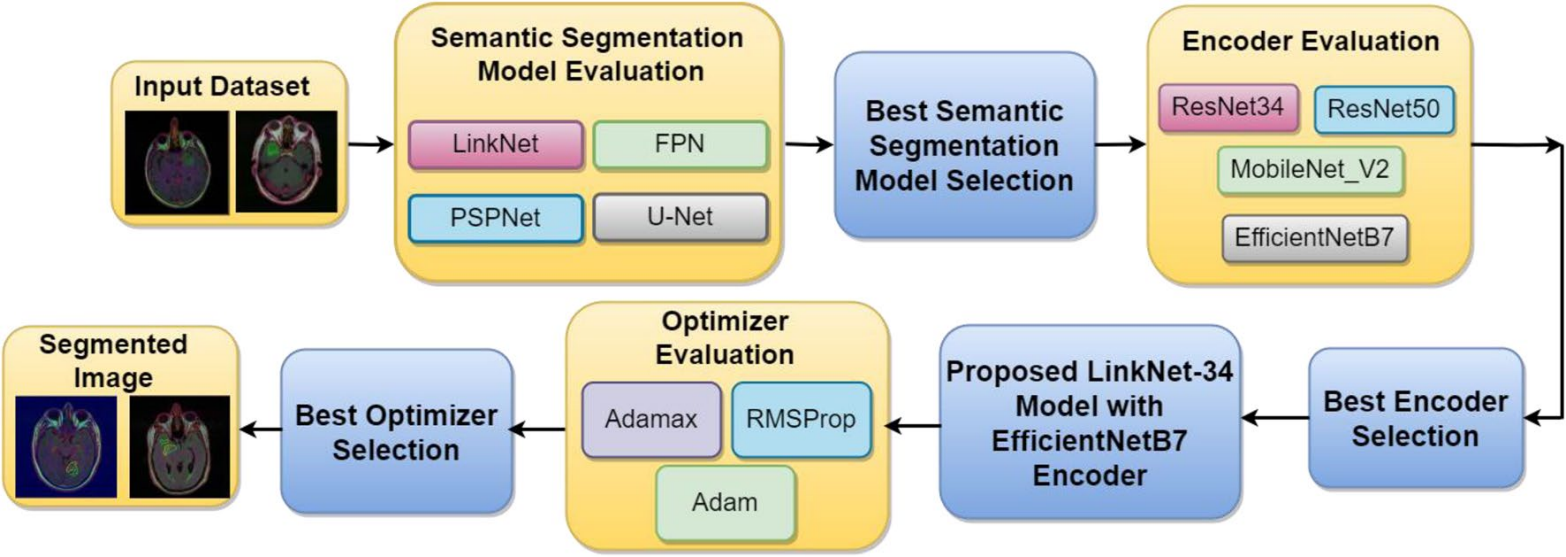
Proposed methodology for segmentation of brain tumor from MRI images.
